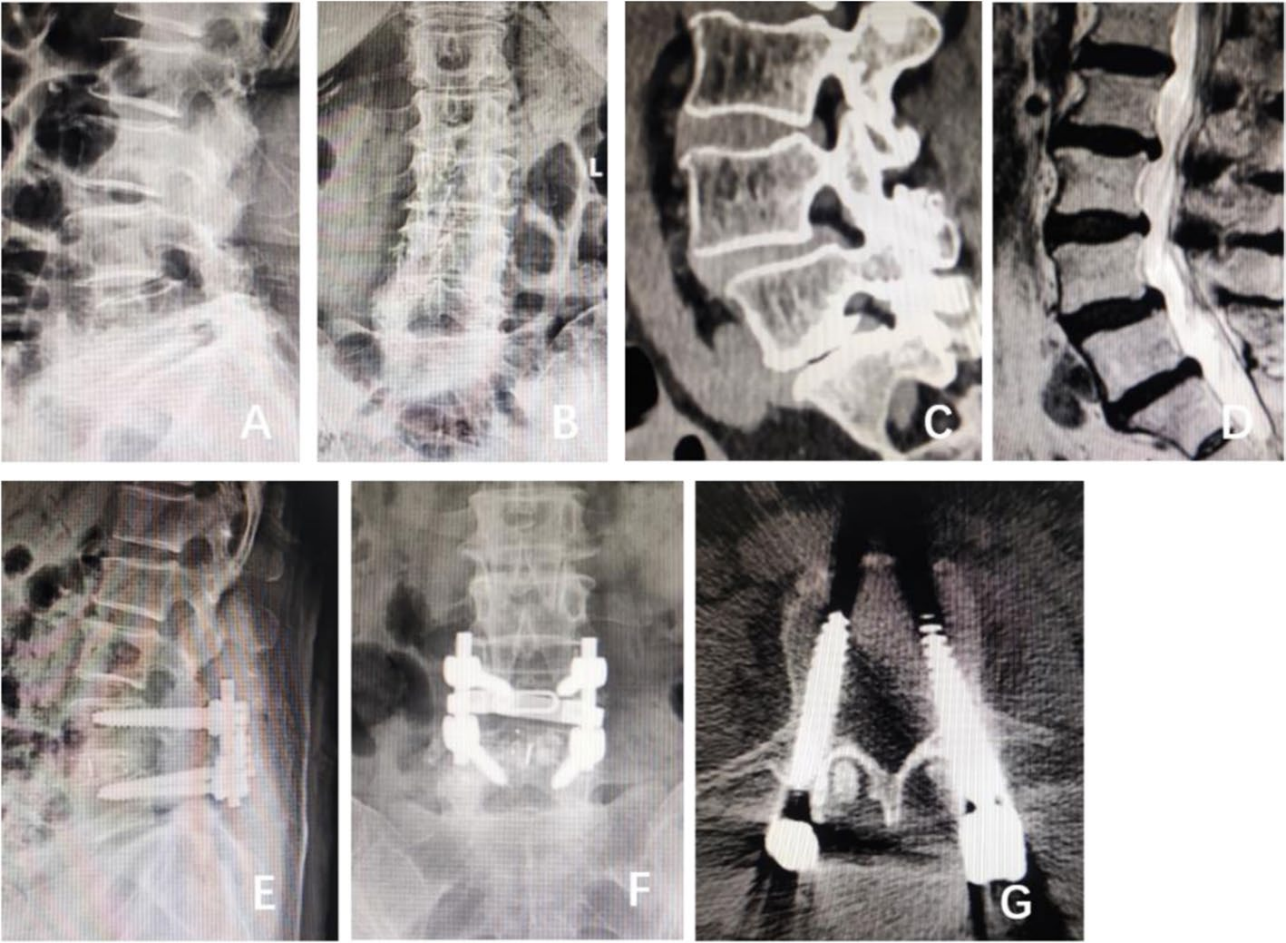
Li Moumou, a 63-year-old female, was diagnosed with degenerative lumbar spondylolisthesis and underwent posterior decompression, bone grafting, fusion and internal fixation at our hospital. A, B: L4 vertebral body spondylolisthesis (I°) before the operation. C: Preoperative CT showing lumbar degenerative changes and L4 spondylolisthesis (I°). D: Preoperative MRI showing L4 spondylolisthesis (I°), degeneration and bulging of L2-3, L3-4 and L4-5 intervertebral discs, and compression of the dural sac at the corresponding levels. E, F: X-ray showing the position of internal fixation of the lumbar spine 2 months after the operation. G: According to Herren’s grading method, the left screw showed no invasion of the proximal facet joint (grade 0); the right screw showed obvious entry of the articular cavity of the proximal facet joint (grade 2)
